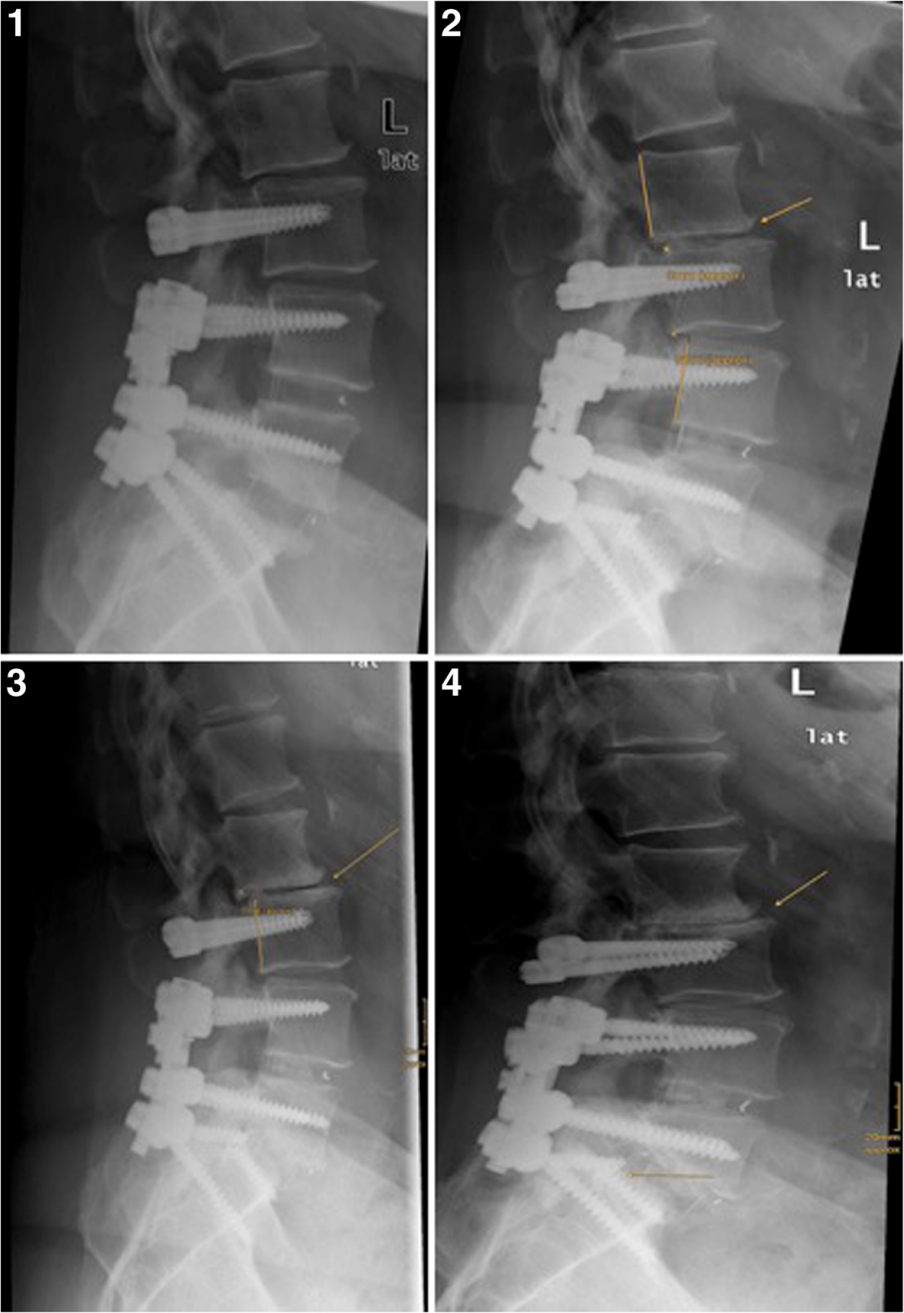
Follow-up of a female patient and onset of radiological detectable ASD without ASDi in 3 steps during 60 months. (1) postoperative plain radiographs of the lumbar spine after PLIF L5/S1 and L4/5 with dynamic instrumentation of L3/4. (2) 12 months follow up: early signs of listhesis, disc space narrowing and beginning spurs formation (L3/4, yellow arrow). (3) 36 months follow up: progressing listhesis and osteochondrosis within the segment superior to the dynamic instrumented segment (yellow arrow). (4) 48 months follow up: listhesis and advanced degeneration of L3/4 (yellow arrow)
